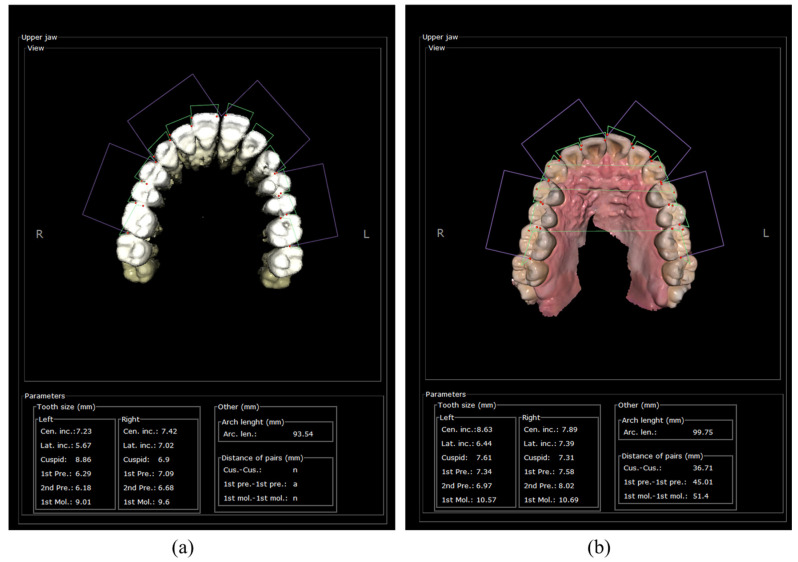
Designed GUI of the developed function for CBCT (a) and the scan images of plaster dental models (b).
